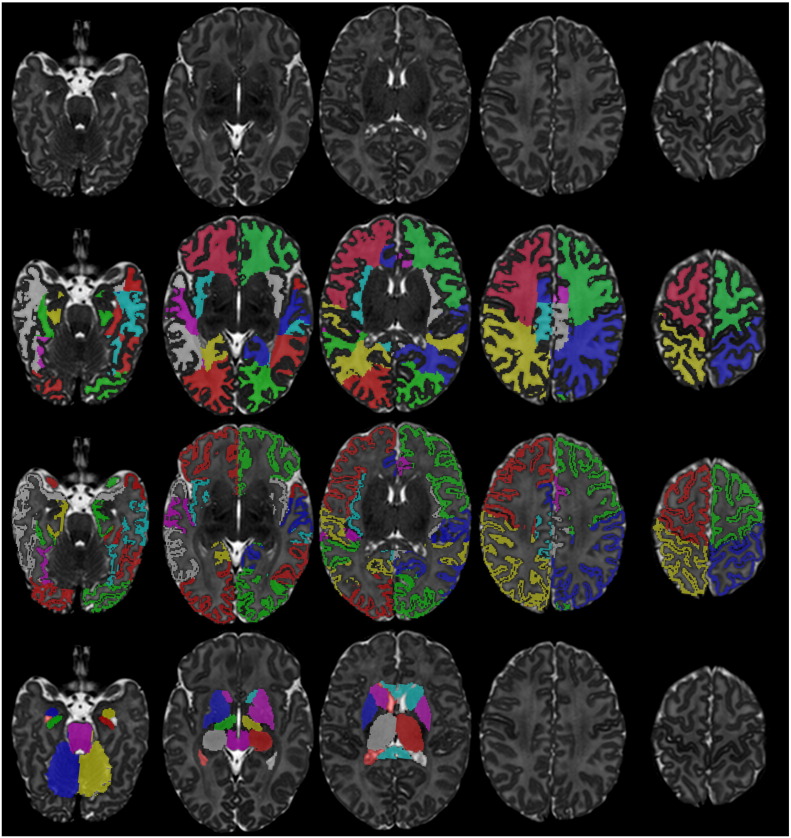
Example segmentation of a neonatal MRI acquired at 44 weeks PMA at scan with the 82 labels overlaid (second row: WM labels, third row: CGM labels, fourth row: subcortical GM labels and ventricles).
